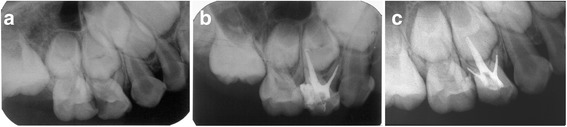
Eighteen months follow-up of MPRCF. a Pre–operative radiograph of 54 considered for root canal treatment with MPRCF. b Radiograph taken immediately postoperatively showing adequate filled. c Radiograph 18 months postoperatively showing the fillings were resorbed at the similar rate with palatal root
